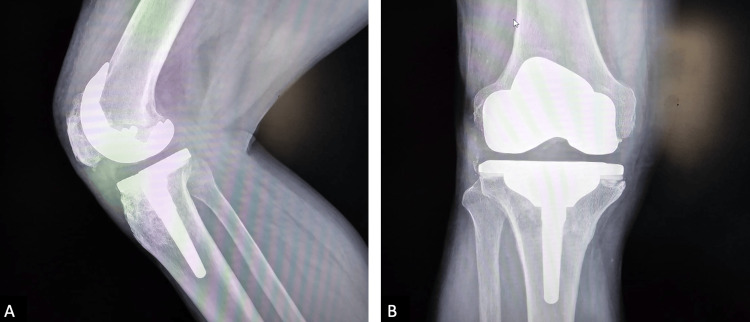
Postoperative X-ray of the right knee joint A: anteroposterior; B: lateral view, and the bone allograft appeared to be intact with minimal remodeling.
